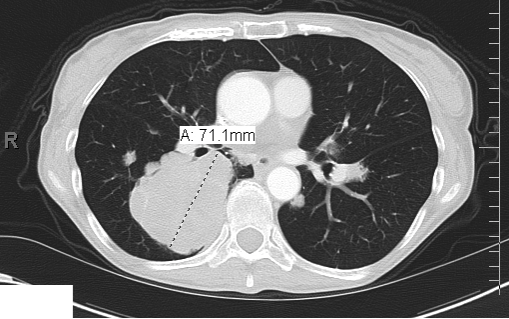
CT scan demonstrating stable disease after five months of sorafenib.
